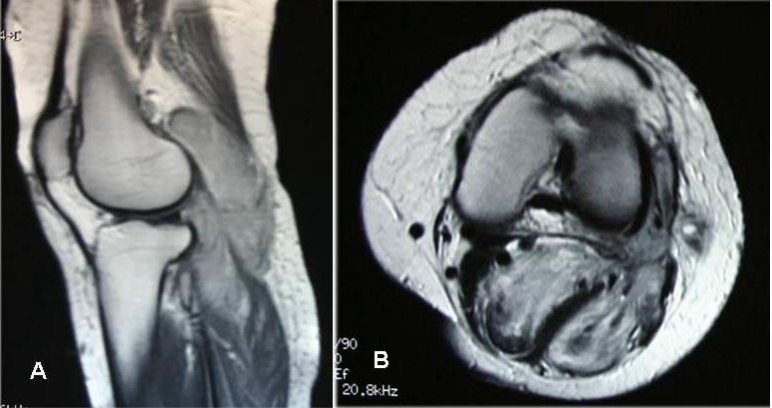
A & B – MR image (longitudinal & transverse sections) of the left knee & leg (Case 2) showing the soft tissue malignant giant cell tumor arising from the muscle posteriorly.
